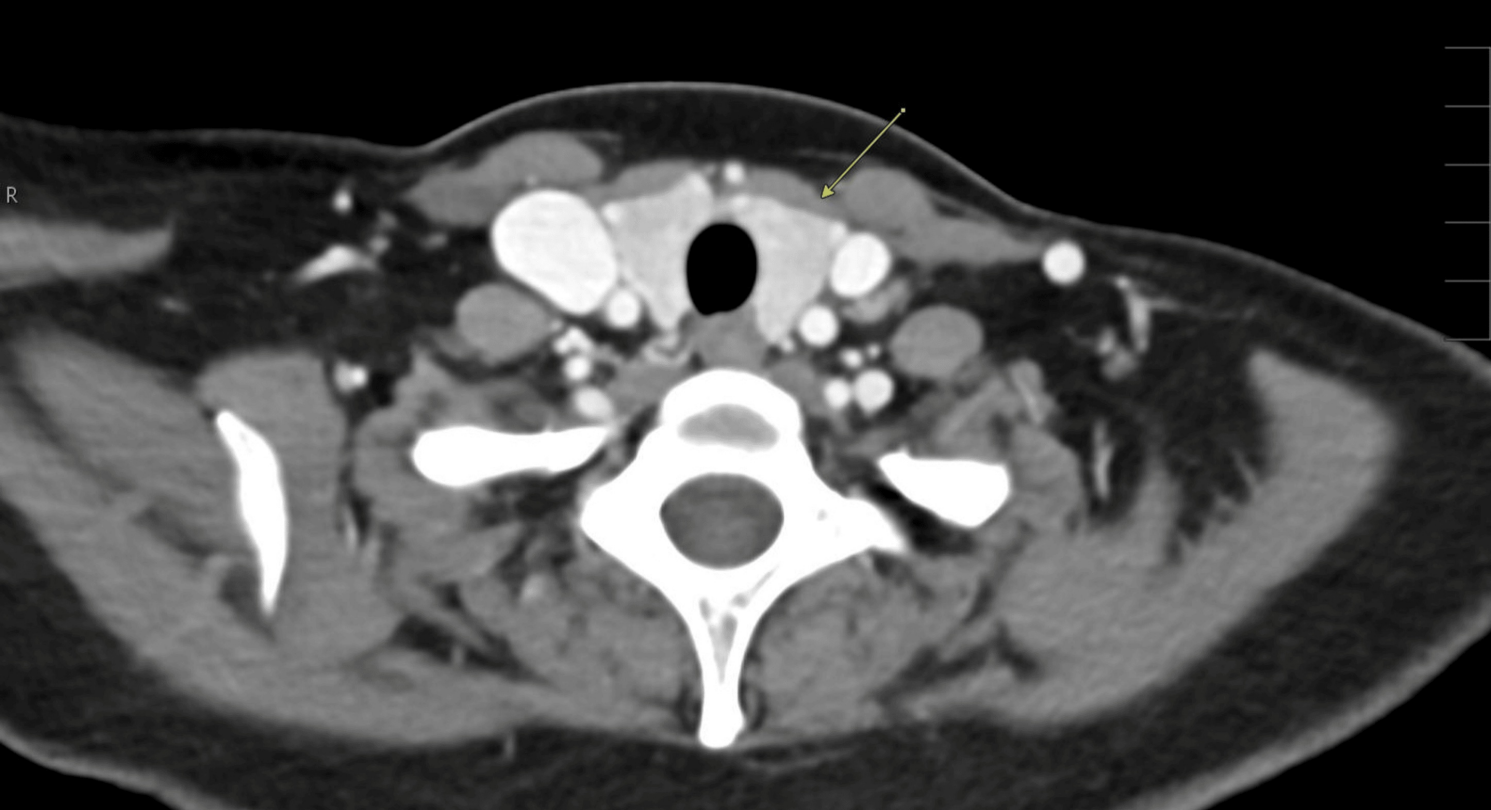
Transverse computed tomography image demonstrating homogeneous appearance of the bilateral thyroid lobes (arrow)
